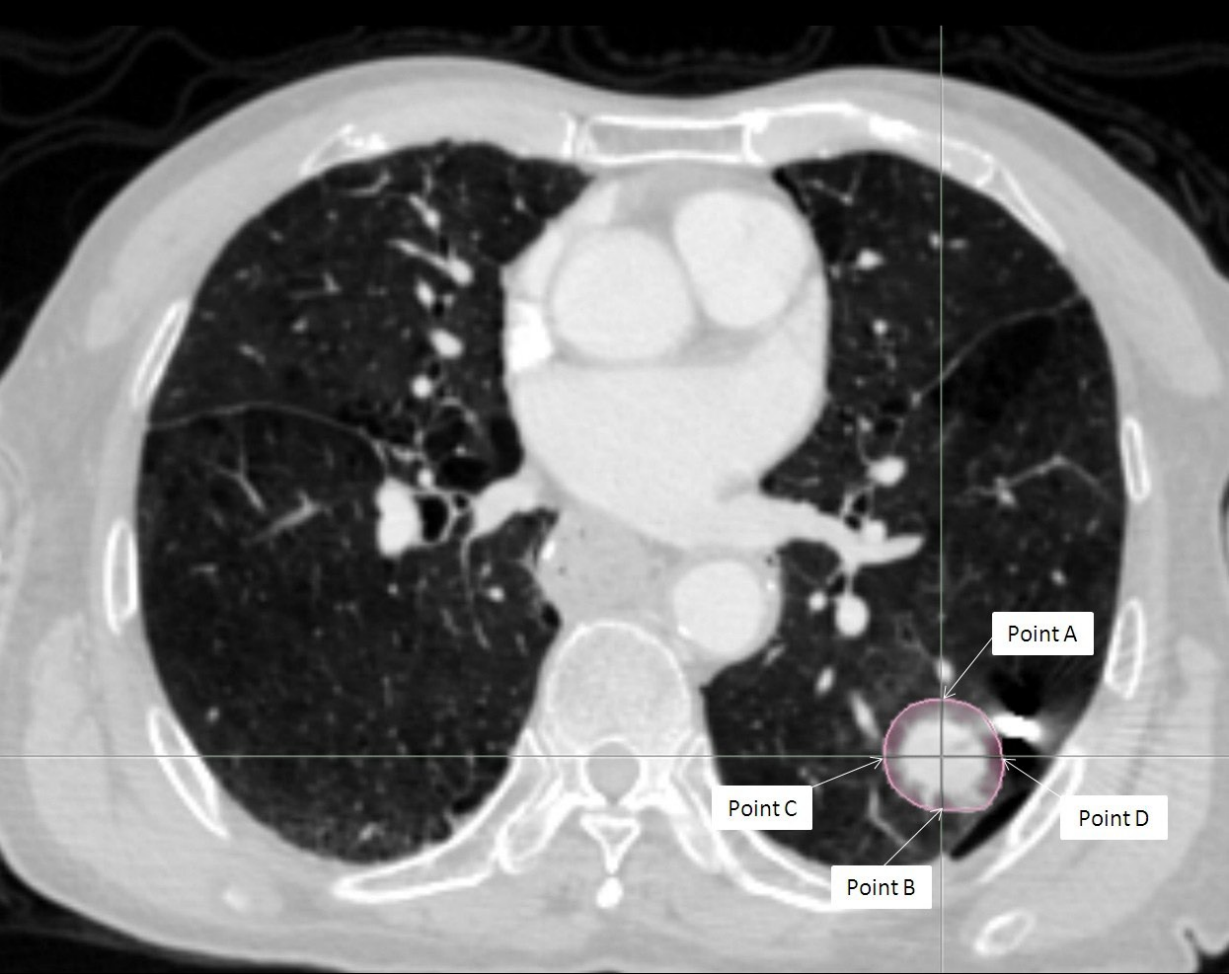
A CT slice at the center of the planning target volume (PTV) showing the locations of the four reference points for a peripheral lung cancer case. Points A, B, and C were situated at the anterior, posterior, and medial borders of PTV, respectively, which were near the soft tissue and lung interface. Point D was at the lateral border, which was in between soft tissues or close to the rib bone.
